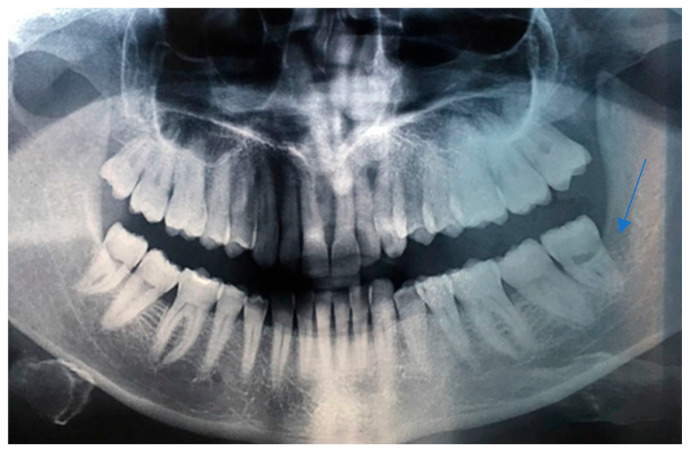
OPG x-ray and the blue arrow show a focus of radiolucency behind the lower-left third molar.
